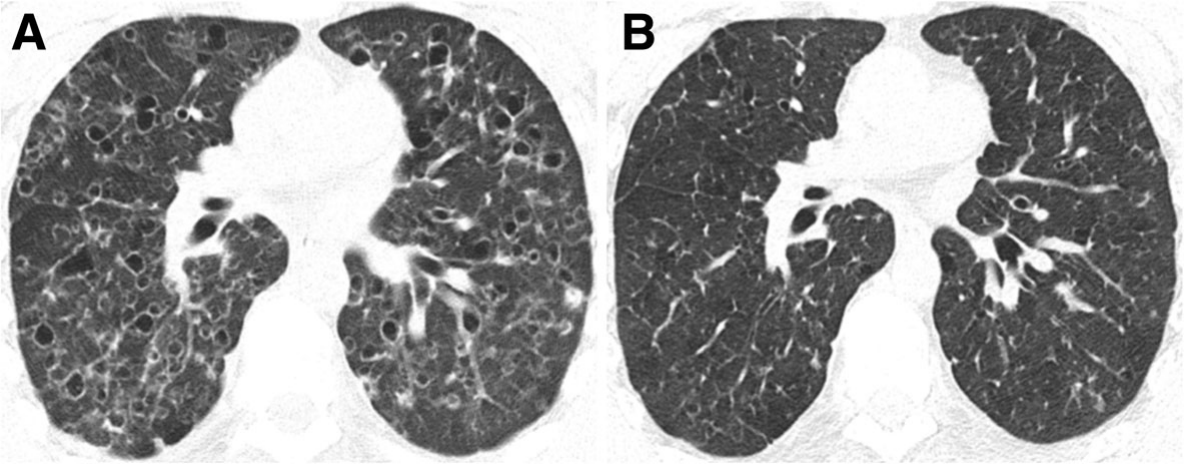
PLCH. Regression of the imaging findings over time (2-year interval), after smoking cessation. a Axial CT image showing cysts with different shapes and sizes diffusely distributed in both lungs. A few cellular and mixed nodules are also present. b Axial CT image at 2-year interval showing almost complete resolution of the imagiological findings
